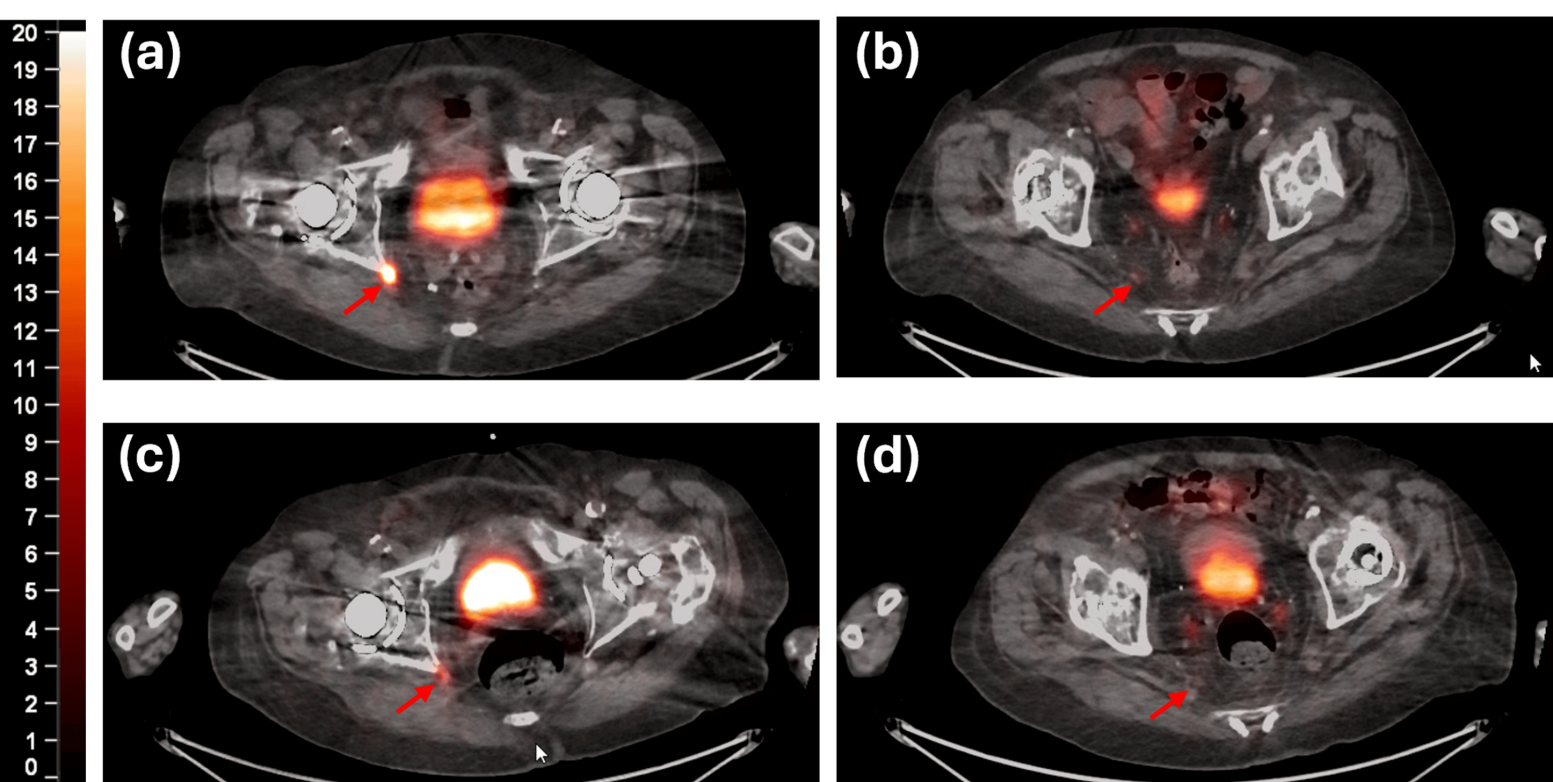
PET/CT images with SUV overlay of pre-treatment: (a) the ischium target (1.8 × 1.3 cm) and (b) the soft tissue perirectal lymph node (0.5 cm). The three-month post-treatment images are presented for (c) the ischium target and (d) the lymph node. PET: positron emission tomography; SUV: standardized uptake value
